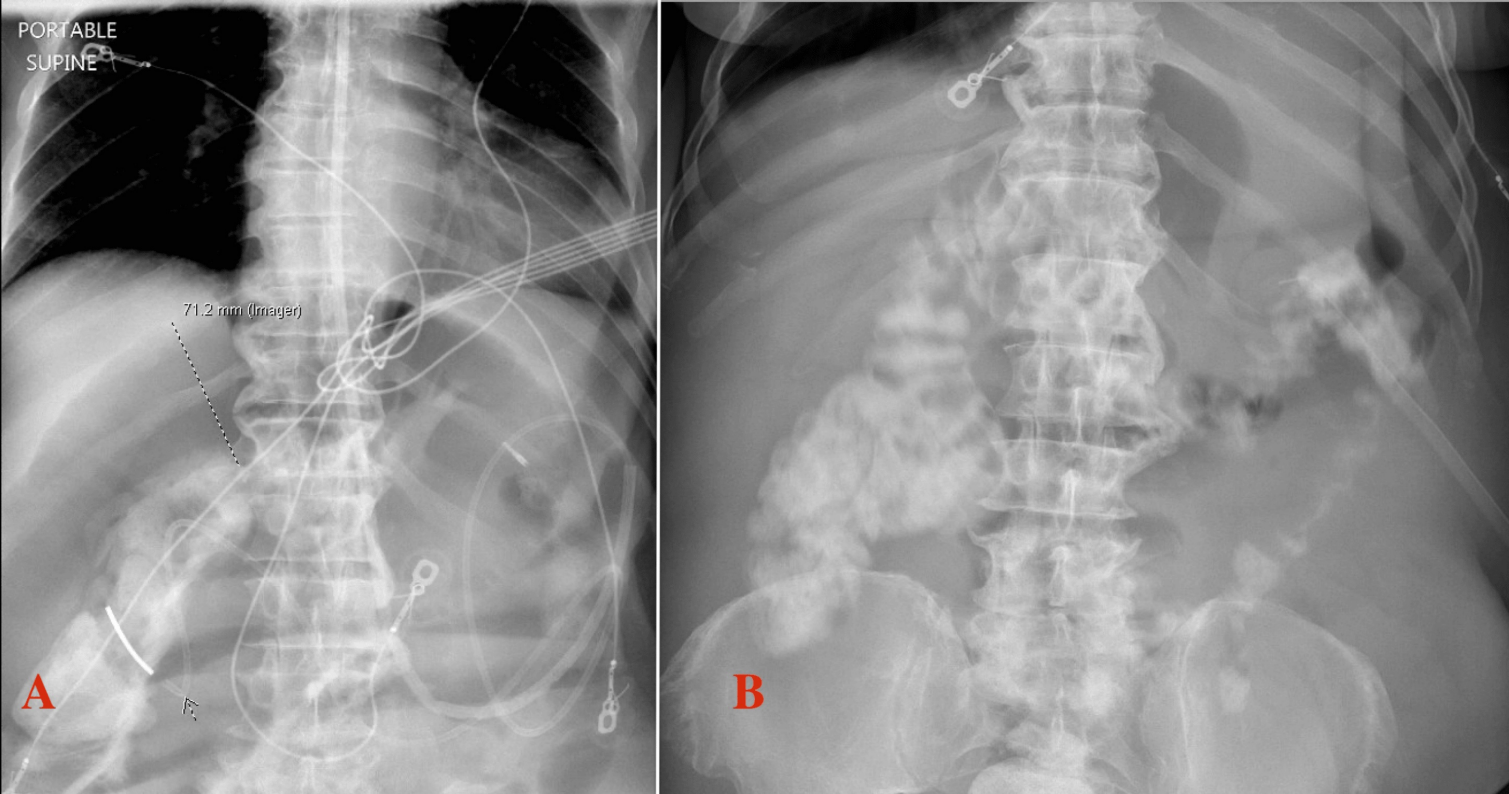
Abdominal X-ray. Abdominal X-ray showing small bowel dilation before (A) and after prucalopride (B). Nasojejunal tube is seen in (A) and percutaneous gastrostomy tube in (B).
